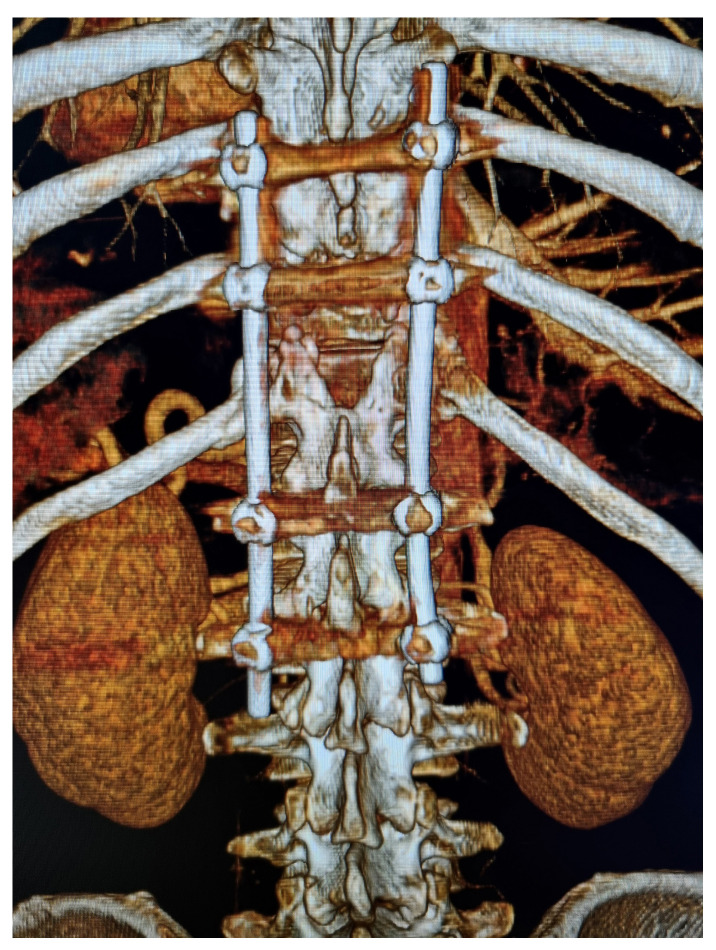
Posterior transpedicular stabilization of the thoraco-lumbar spine: CT reconstruction.
